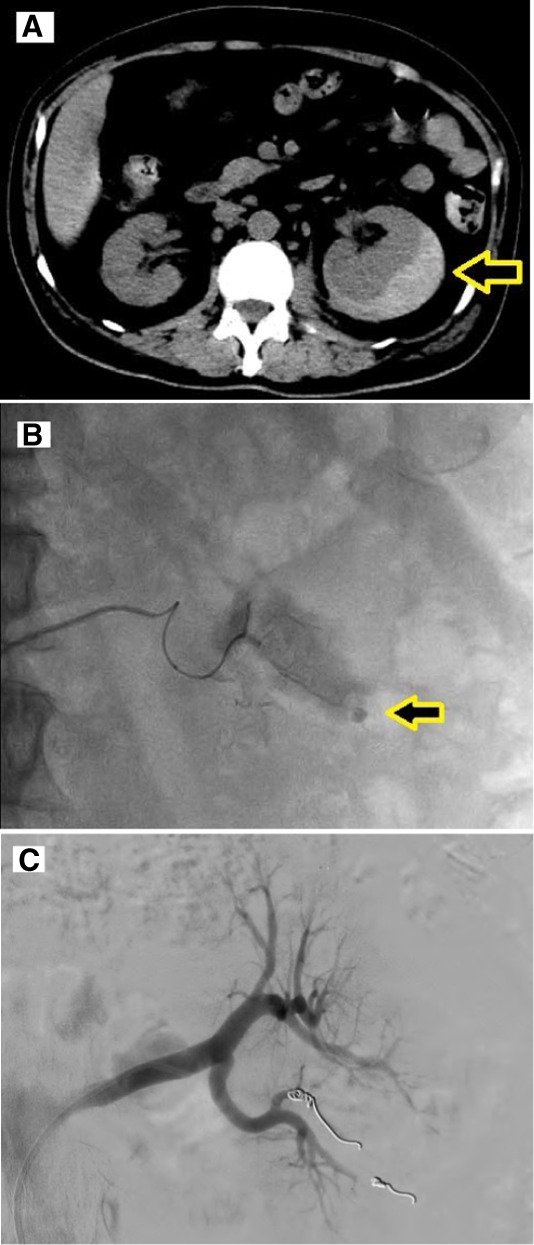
Radiological confirmation and embolization of spontaneous renal hemorrhage. (A) Noncontrast CT showing a crescent-shaped left-sided subcapsular perirenal hematoma (arrow). (B) DSA showing focal contrast extravasation from a lower pole segmental artery (arrow). (C) Postembolization angiogram showing successful occlusion of the segmental artery.
